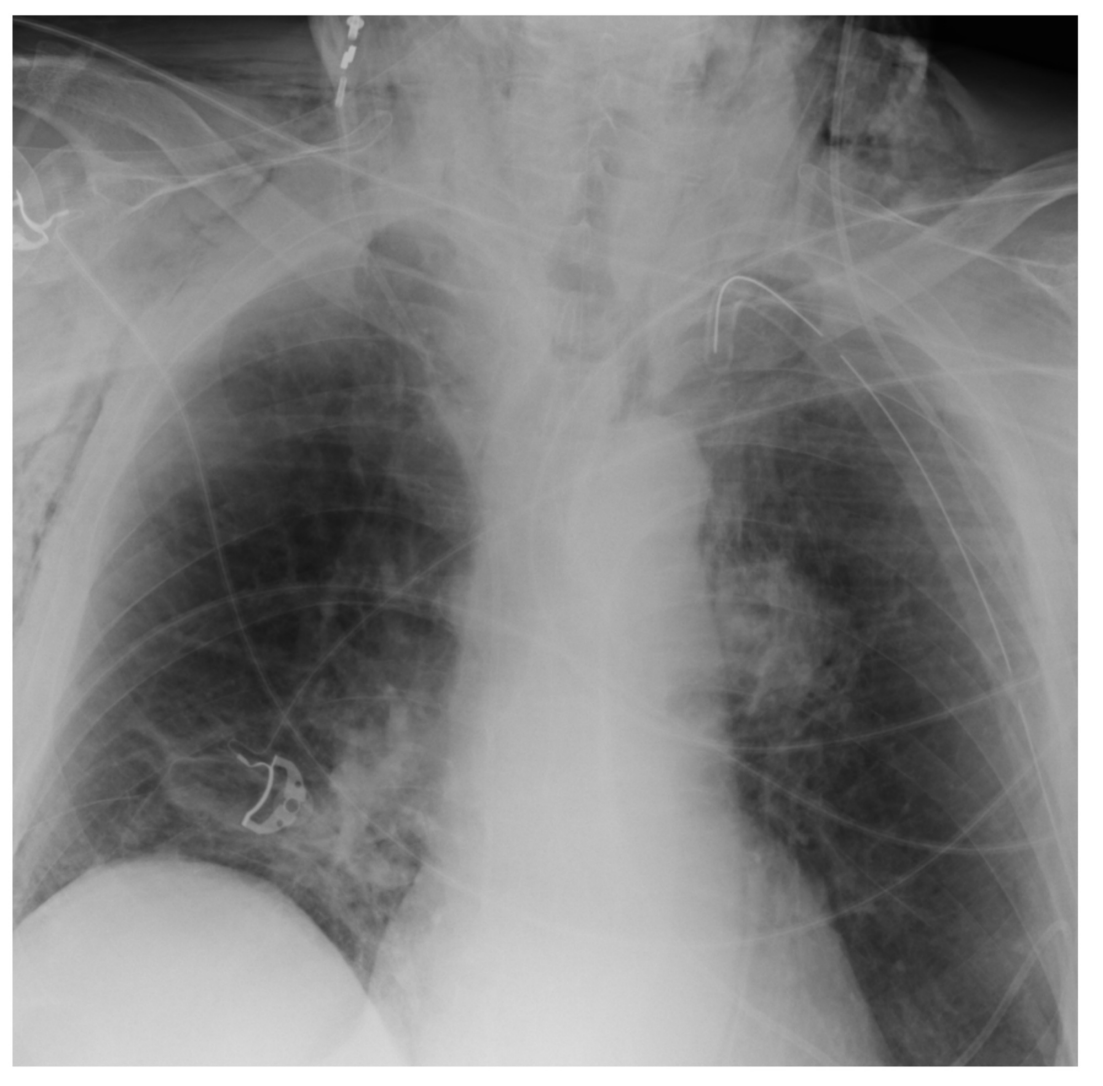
Postoperative chest X-ray after VATS wedge resection of the cranial part of the left upper lobe. VATS, video assisted thoracoscopic surgery
